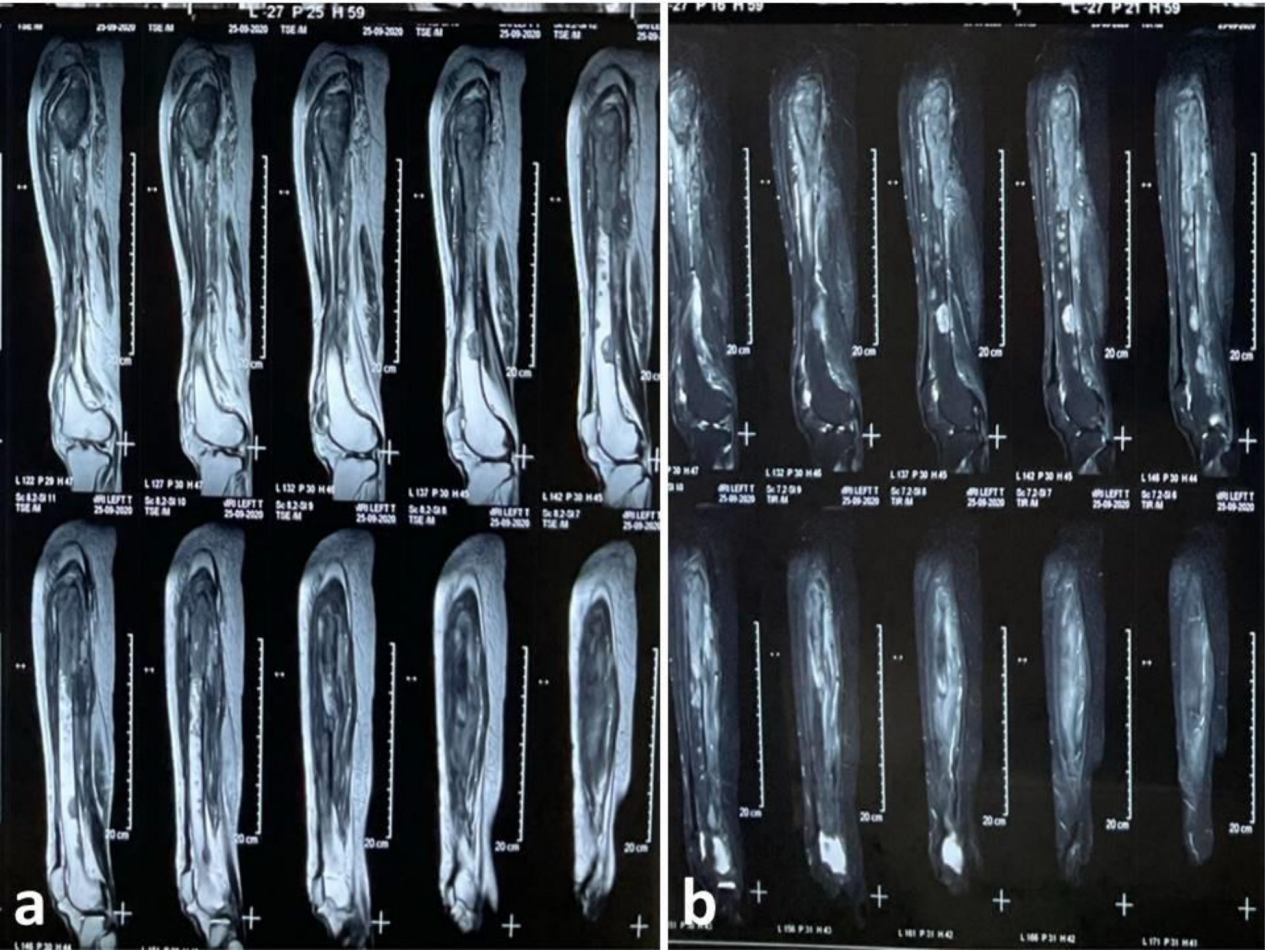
Magnetic resonance imaging sagittal images of the left thigh show a large heterogeneous T1-weighted (a) and fat-suppressed/ short tau inversion recovery (STIR) (b) hyperintense lesion involving the left proximal femur, neck, intertrochanteric region, and proximal shaft with areas of cortical break. Multiple other round lesions are present in the femur’s mid and distal shaft.
